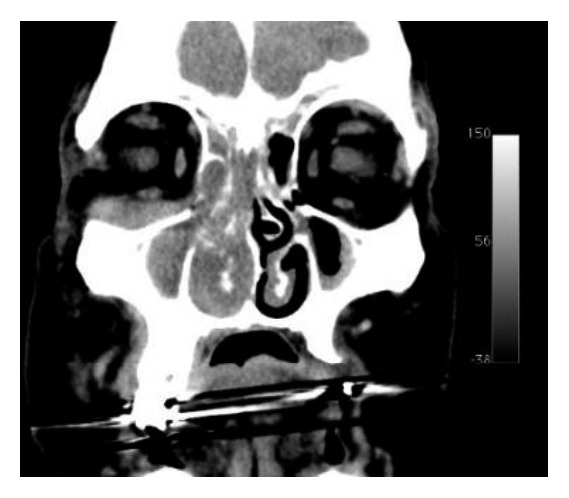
CT demonstrates lesion occupying ethmoid cells, nasal cavity, and maxillary sinus and extending through the lamina papyracea.
